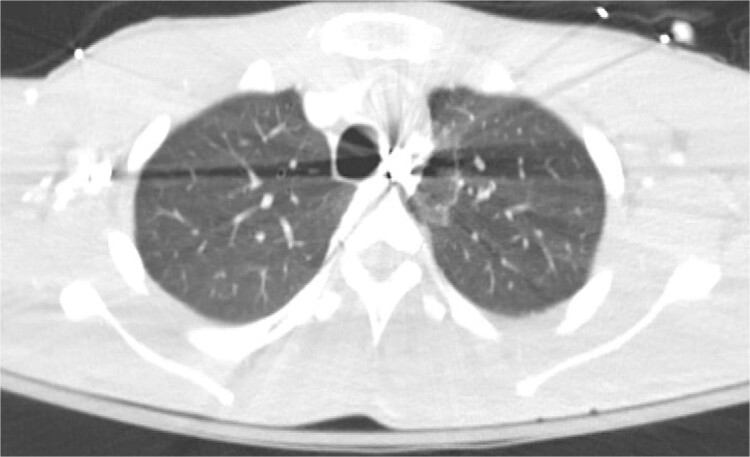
CT angiogram of chest showing retained projectile.
